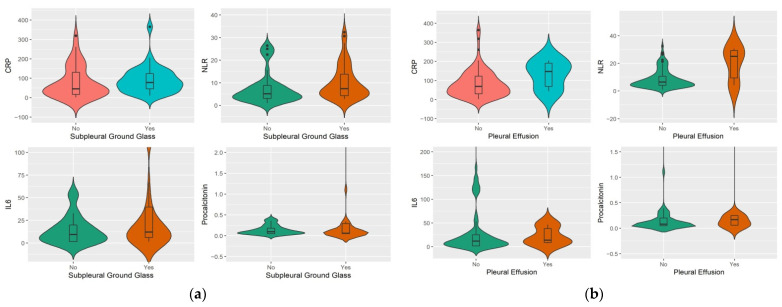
Difference in medians of inflammatory markers by presence on CT: (a) subpleural ground glass; (b) pleural effusion.
